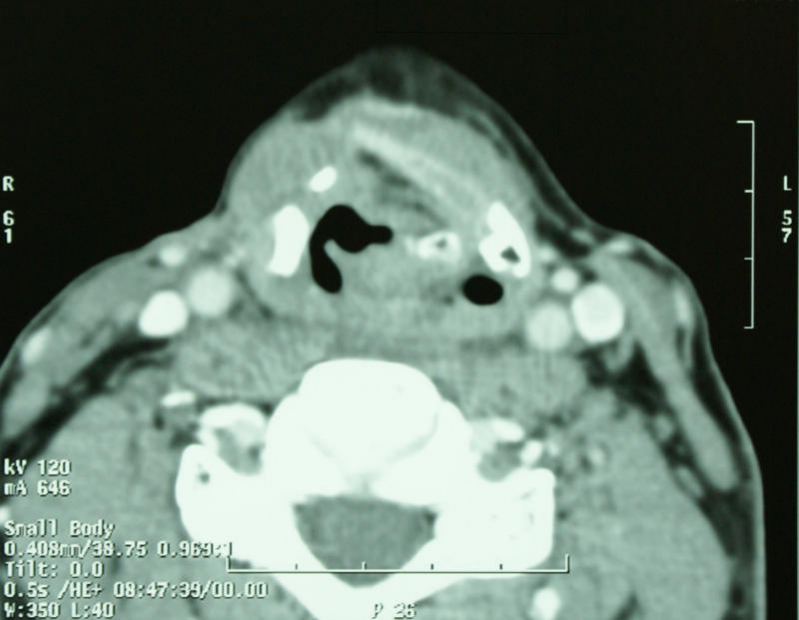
A two-phase CT scan, performed in March 2009 showing the postoperative thyroid cartilage defect on the right side. Soft tissue mass protruding into the supraglottic region on the left side in the aryepiglottic fold is identical to changes described in 2007 and 2008 (no signs of recurrence).
